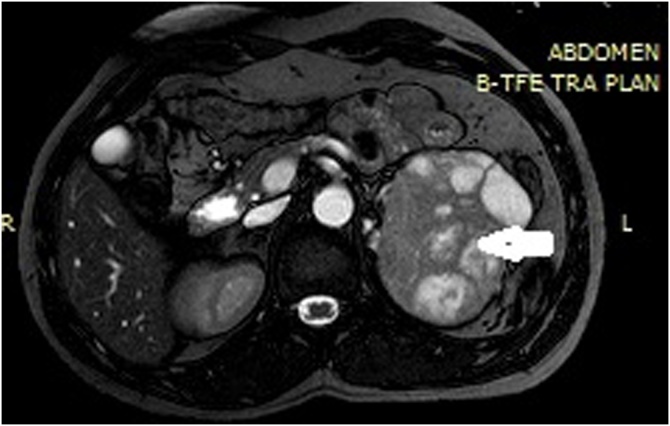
Axial sections of MRI abdomen showed the hetrogenous mass in the left adrenal gland(arrow).
